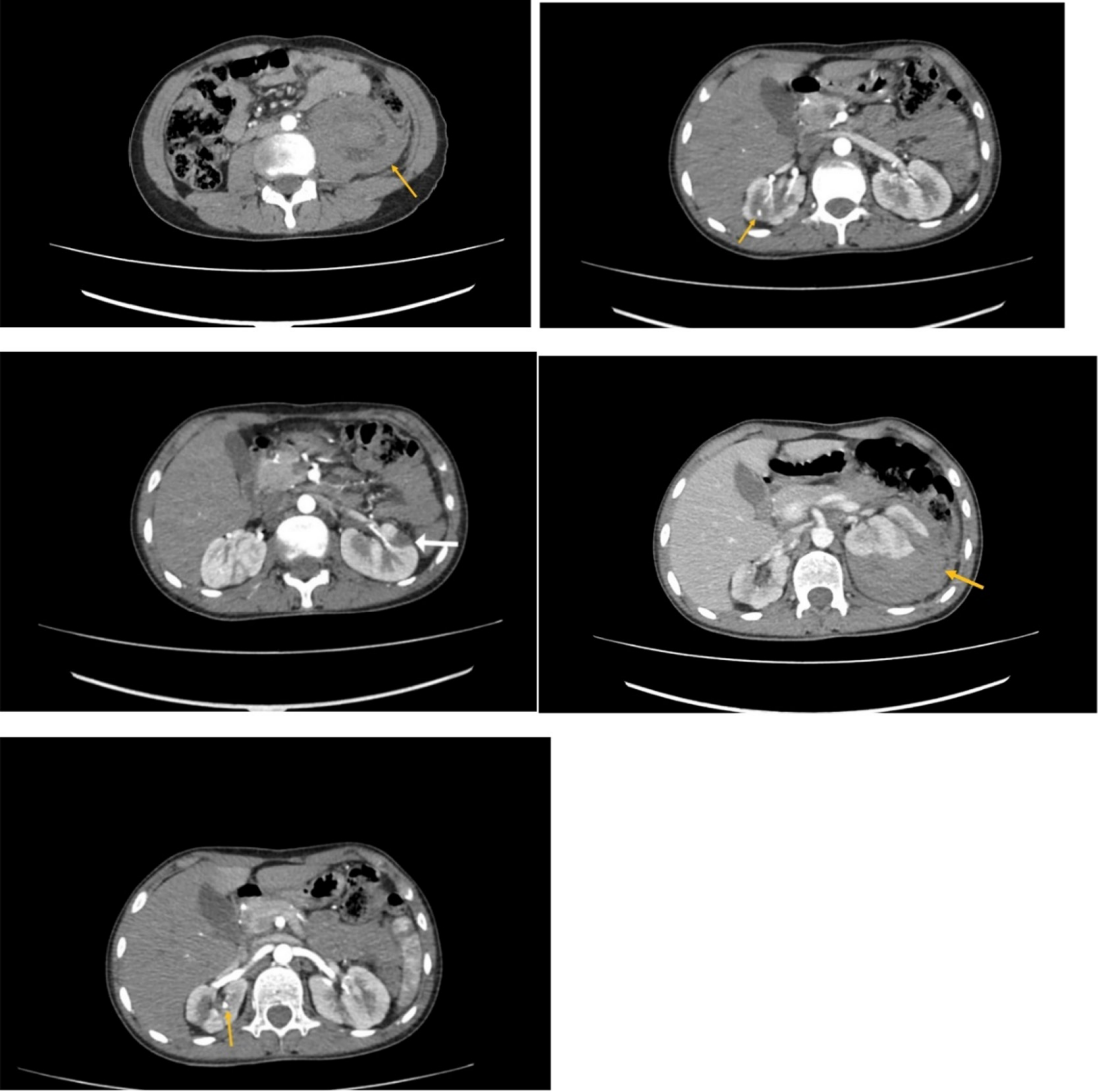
Axial CT scan of abdomen with retroperitoneal hemorrhage with compression of left kidney previous infarct and no evidence of aneurysms.
